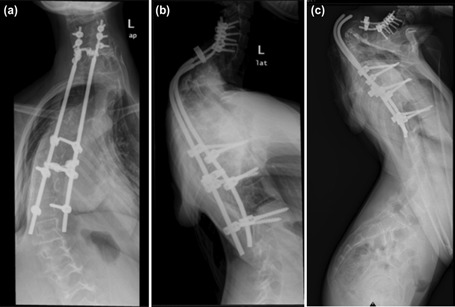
(a, b) Radiographs of the spine showing posterior spinal instrumentation and fusion in situ. (c) Broken rods at the cervicothoracic junction one year later.
